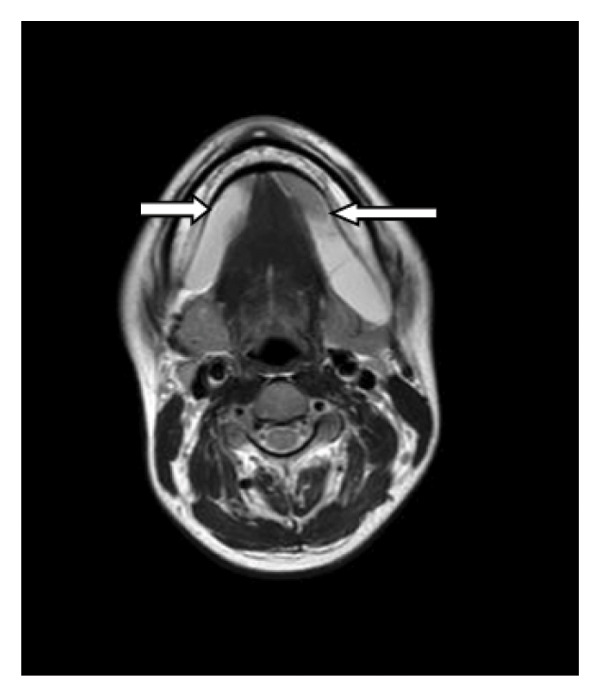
MRI cross‐sectional images reveal bilateral cystic masses in the mandible, extending into the submandibular space. The masses exhibit well‐defined borders and show no enhancement.
